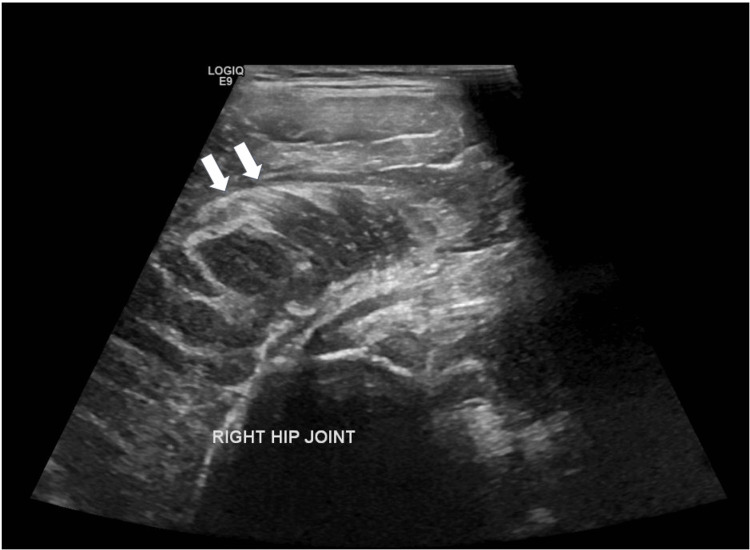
B-mode ultrasound of the right hip joint demonstrating a bulky gluteus minimus muscle (white arrows) with disorganized muscle fiber architecture.
